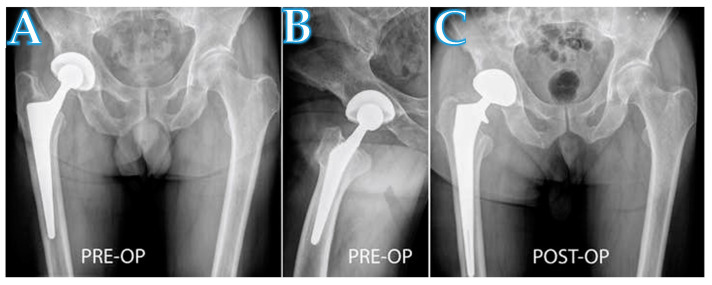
A radiograph showing a patient with aseptic loosening of a femoral stem in the right hip (A,B). The implantation of the long revision stem was performed with a proximal extension of the DAA (C).
